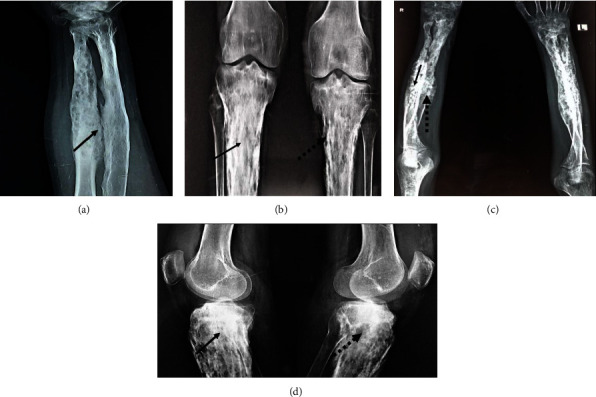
(a) X-ray right forearm (both radius and ulna) showing hyperostosis with few lytic area, (b) X-ray knee (AP) showed bilateral tibia periosteal reaction, hyperostosis, osteolysis with osteopenia, (c) Lytic lesions and hyperostosis increased after 1 year during replace, (d) increased sclerotic lesion, lytic lesion with osteopenia after 1 year during relapse. Plain arrow-sclerotic lesion, dotted arrow-lytic lesion.
